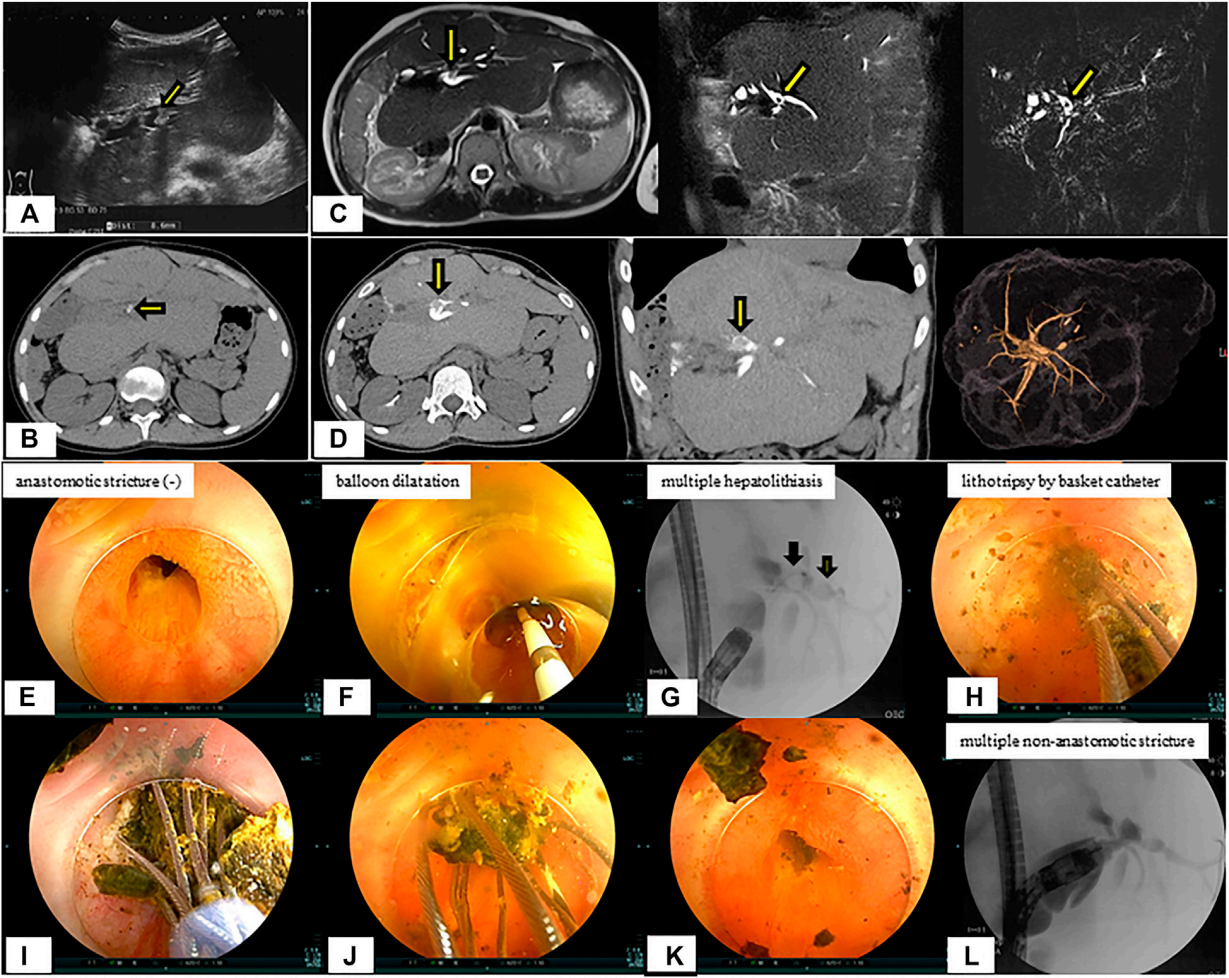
Radiological findings and endoscopic lithotripsy for hepatolithiasis in a patient with multiple non-anastomotic biliary strictures. (A) Hepatolith (diameter 8.6 mm) with acoustic shadowing on ultrasonography was observed at the confluence of the biliary tracts of Segments 2 and 3. (B) Hepatolithiasis with a high density lesion on computed tomography scan was observed at the confluence of the biliary tracts of Segments 2 and 3. (C) Magnetic resonance imaging. (D) Drip infusion cholangiographic-computed tomography. (E) Anastomotic biliary stricture was not observed by double-balloon enteroscopy. (F) Balloon dilatation for intrahepatic bile duct stricture was performed. (G) Multiple hepatoliths were demonstrared on cholangiography at the confluence of the biliary tracts of Segments 2 and 3. (H–K) Lithotripsy by basket catheter was performed. (L) Multiple intrahepatic bile duct strictures on cholangiography were observed and hepatoliths disappeared.
